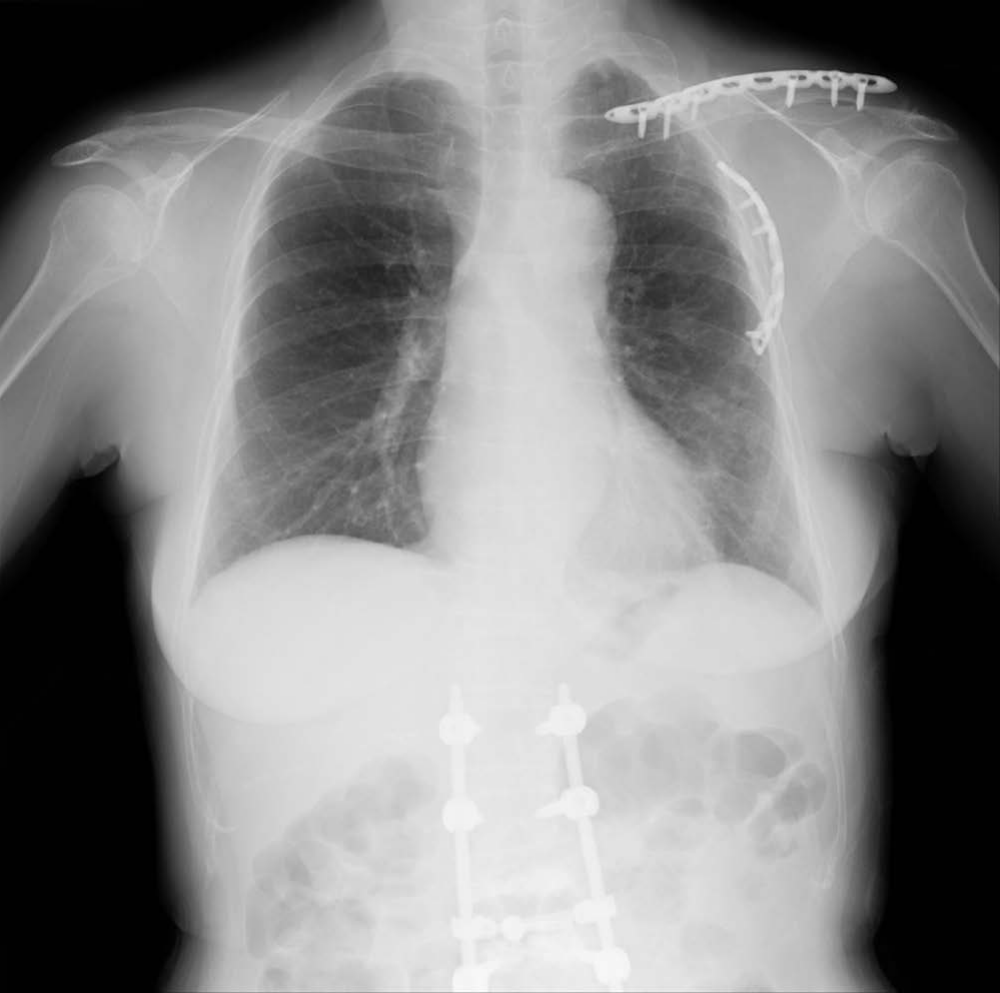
Chest radiograph at discharge shows good general condition of the chest wall, clavicle, and lumbar spine.
